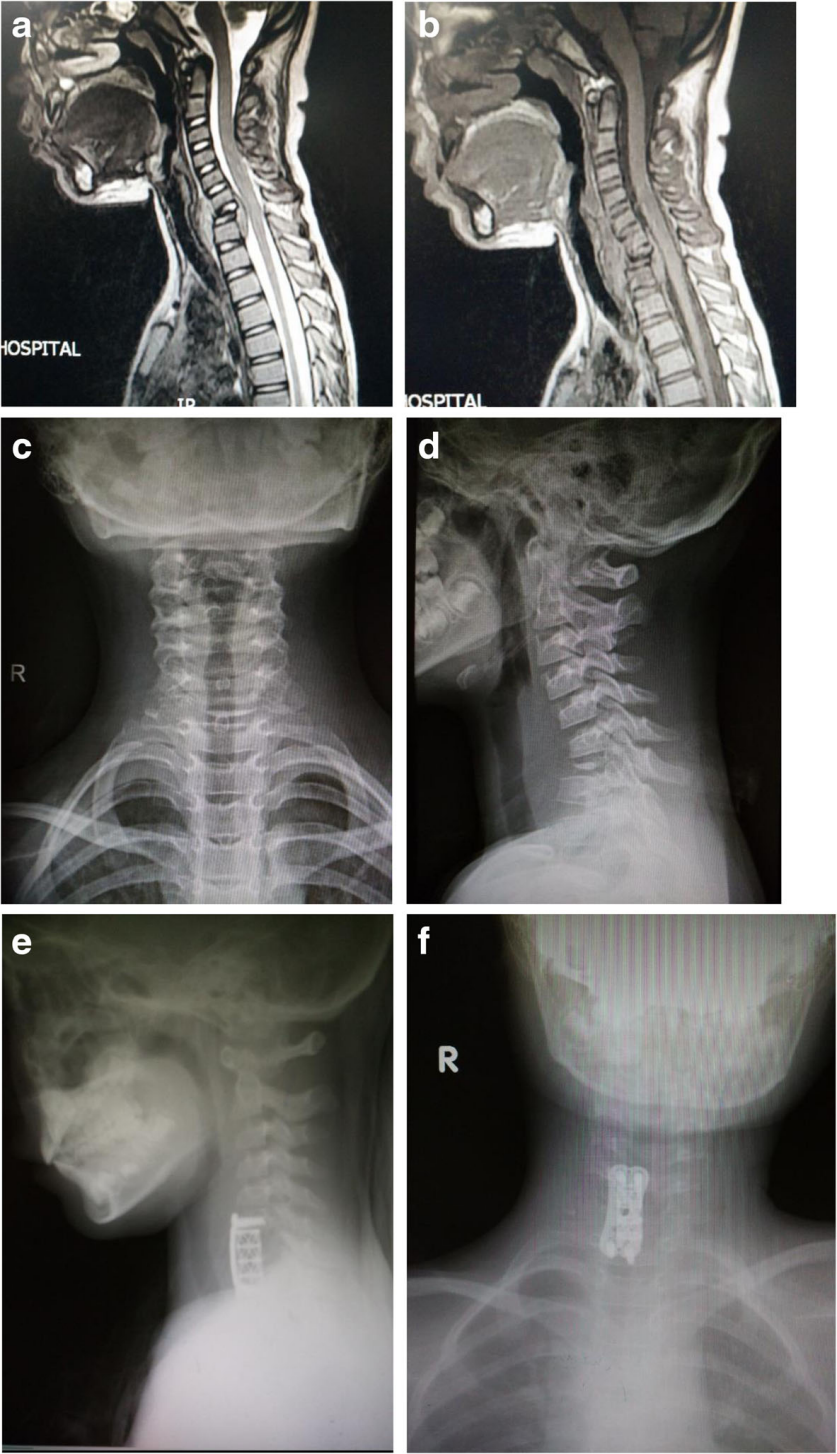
Case 4: a 7-year-old girl, C7 LCH. a, b T1- and T2-weighted MRI images before operation. c, d Anterioposterior and lateral plain film before operation. e, f Anterioposterior and lateral plain film 15 months after operation of anterior tumor resection and miniplate and screw instrumentation
